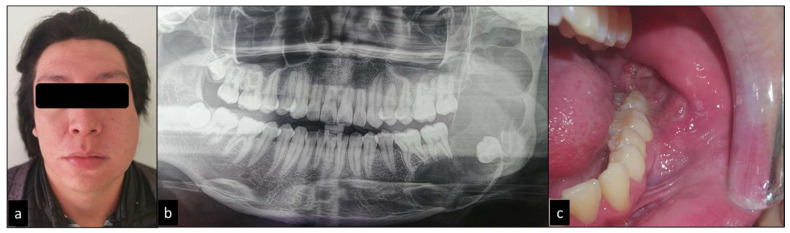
Clinical image. (a) Extraoral image shows large and diffuse swelling at middle and lower left facial third causing facial asymmetry. (b) Panoramic radiograph image showing a multilocular radiolucent area located in the left mandibular body that extends from the mesial root of the lower left first molar to the ramus of the mandible, with retention of the third molar. (c) Intraorally showed expansion of the lingual and vestibular cortices, as well as a polypoid projection in the retromolar area.
